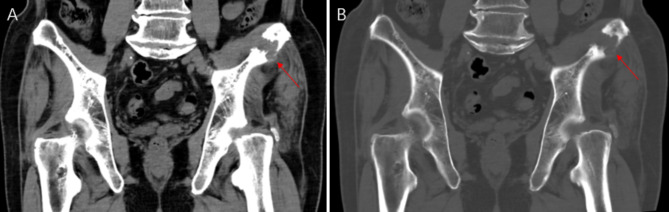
CT revealed a lesion of osteolytic bone destruction in the left ilium with a soft tissue mass (A, arrow). The bone cortex was damaged and reactive osteosclerosis can be seen around the lesion (B, arrow)
